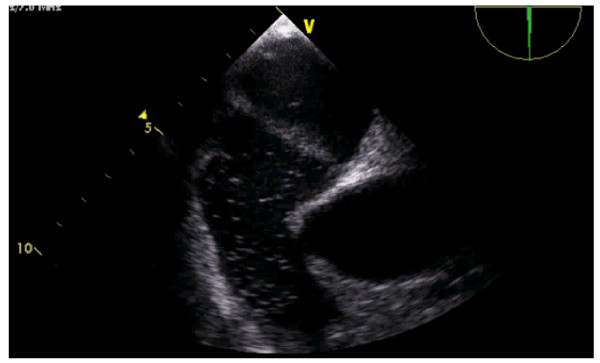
Transesophageal echocardiography. The injection of bolus of agitated saline in a forearm vein confirmed a small PFO, with spontaneous shunt. Note the small amount of bubbles (less than 5) in the left atrium.
